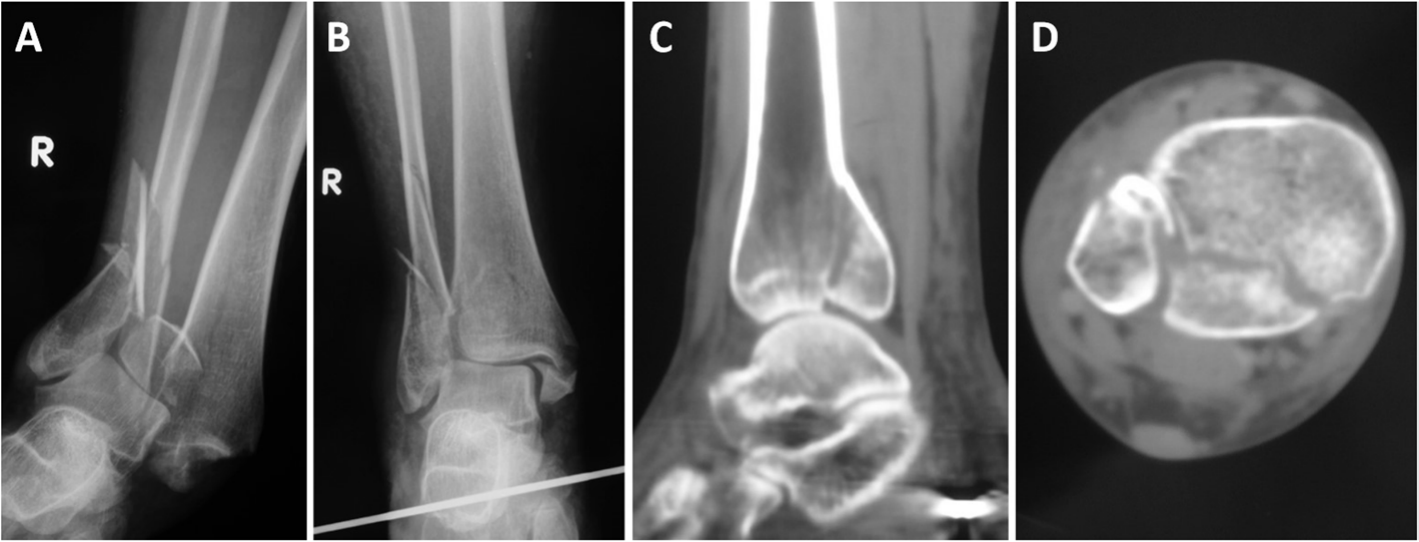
Open Logsplitter injury of the right ankle joint. A,B Anteroposterior X-ray film of the right ankle before and after reduction. C,D Logsplitter injury combined with posterior compression fracture of the distal tibia as displayed by CT of the right ankle joint (posterior Pilon fracture)
